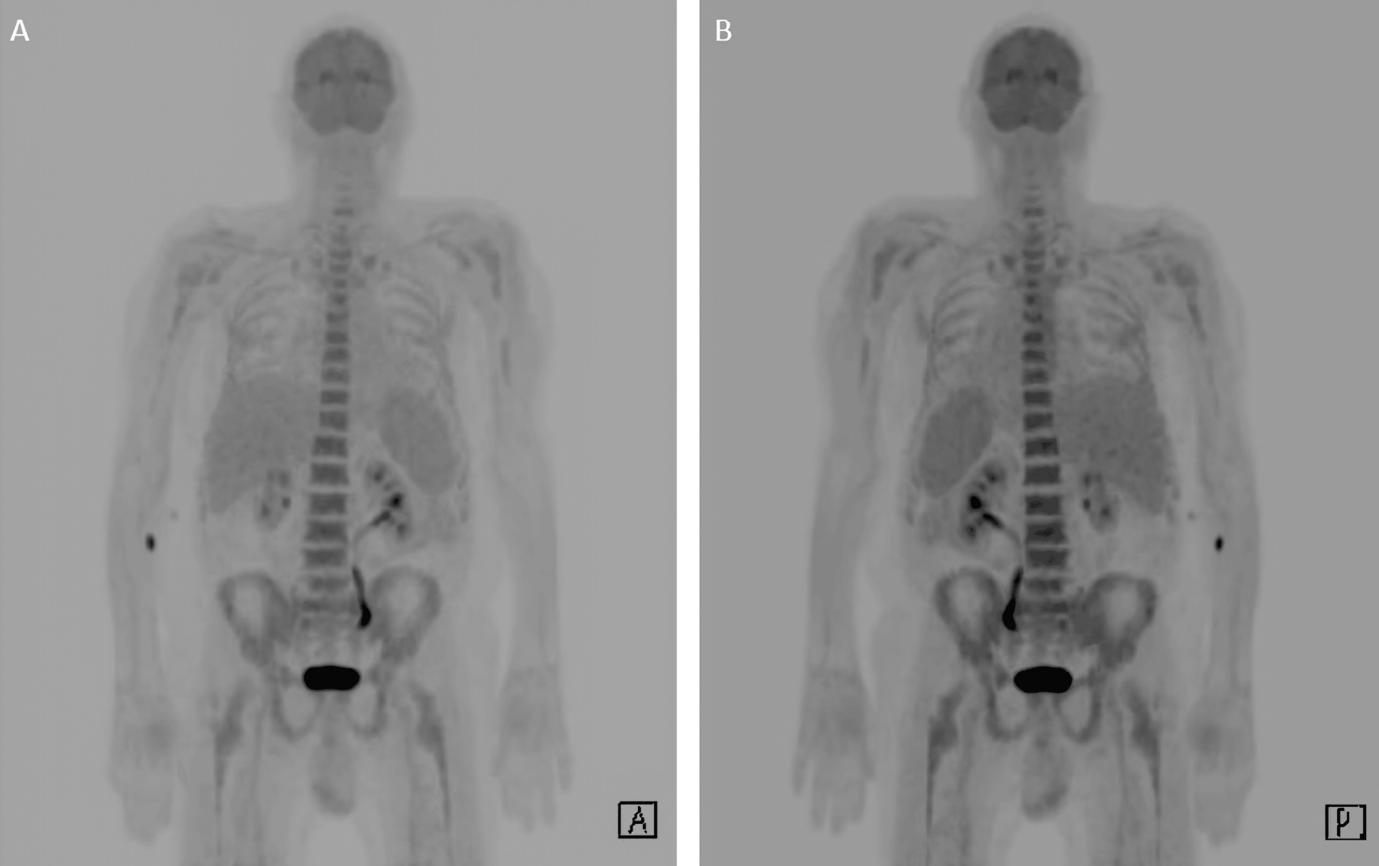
FDG PET-CT MIP series from anterior (A) and posterior (B) perspectives, demonstrating increased uptake within the patient’s bone marrow and spleen. FDG, fluorodeoxyglucose; PET, positron emission tomography; CT, computed tomography; MIP, maximum intensity projection
